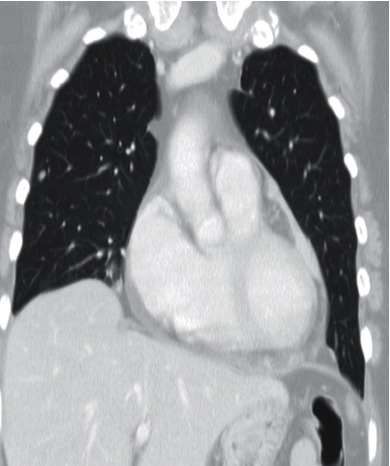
Thoracic computed tomography showing the presence of pericardial effusion.
